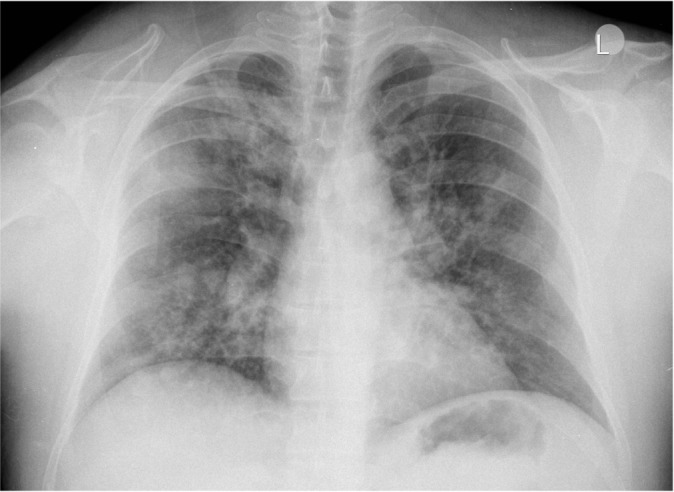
Multiple infiltrates on a chest x-ray
